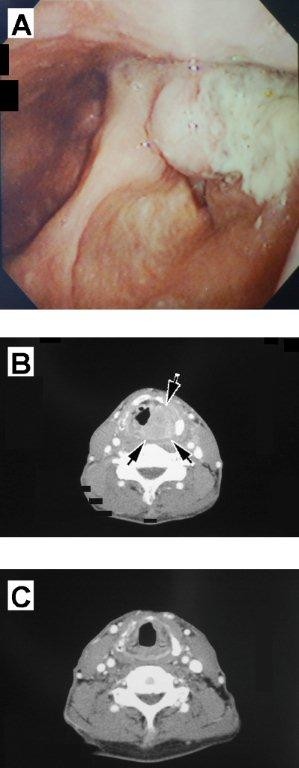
Before and after chemoradiotherapy. (a) Fiberoptic endoscopy showing a neoplastic lesion in the left pyriform recess. (b) CT showing a tumor occupying the left pharyngeal cavity and infiltrating the thyroid cartilage. (c) CT performed after the completion of chemoradiotherapy, showing no evidence of neoplastic lesions.
